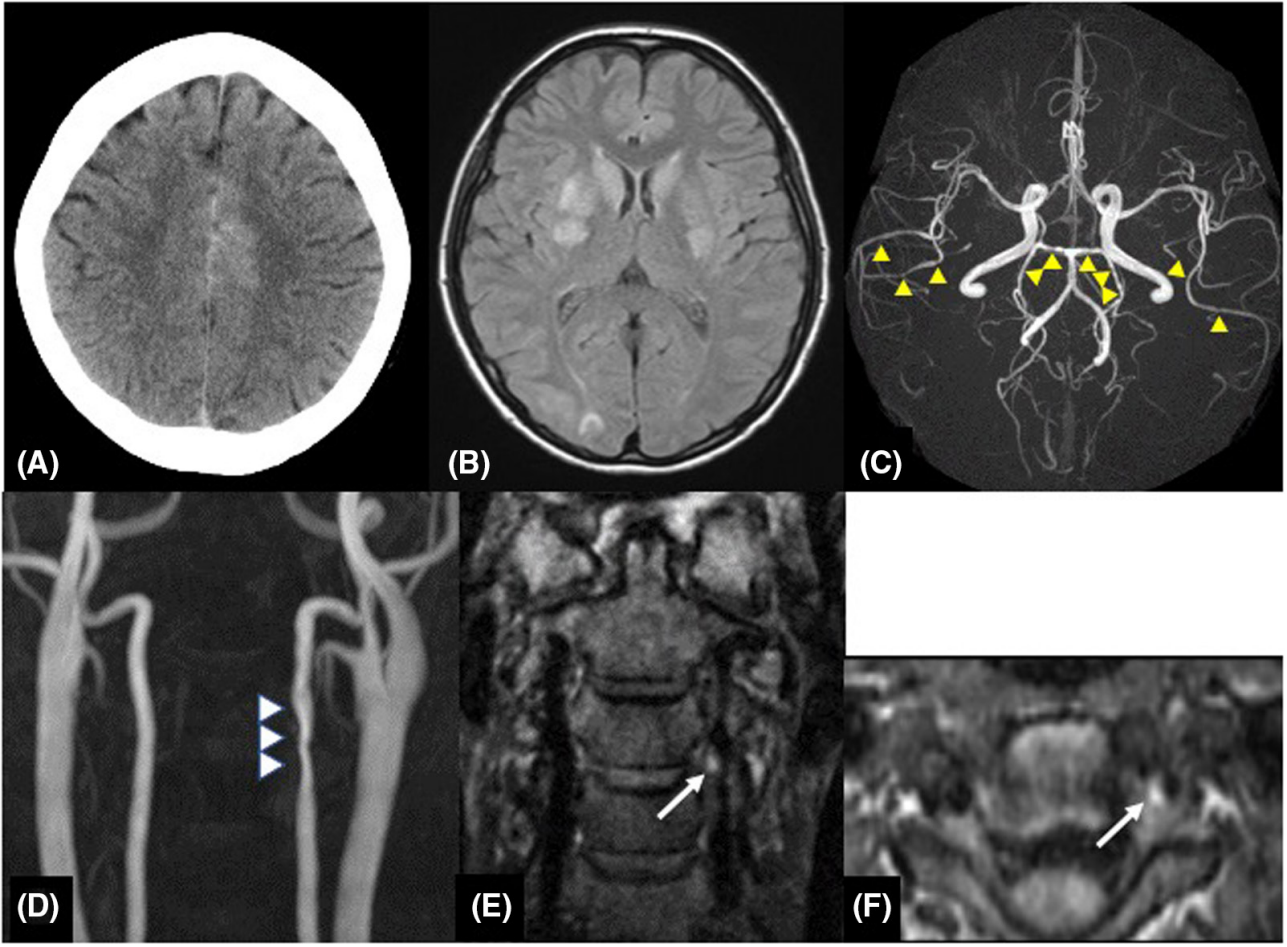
Neuroradiological findings at onset. (A) Plain CT scan revealed hyper density along the right cingulate sulcus, consistent with SAH. (B) MRI FLAIR images revealed hyperintensity involving the right occipital lobe and bilateral basal ganglia. These changes represent vasogenic edema due to PRES. (C) Magnetic resonance angiography (MRA) showed segmental narrowing (yellow arrowheads) of the bilateral middle and posterior cerebral arteries. (D) In right extracranial vertebral artery at the C3/4 level, cervical MRA (3D Time‐of‐flight) showed vessel contour abnormality (white arrowheads) and MSDE coronal (E) and axial (F) images showed a periluminal rim (white arrow) indicative of an intramural hematoma (IMH) suggesting a possible of dissection
